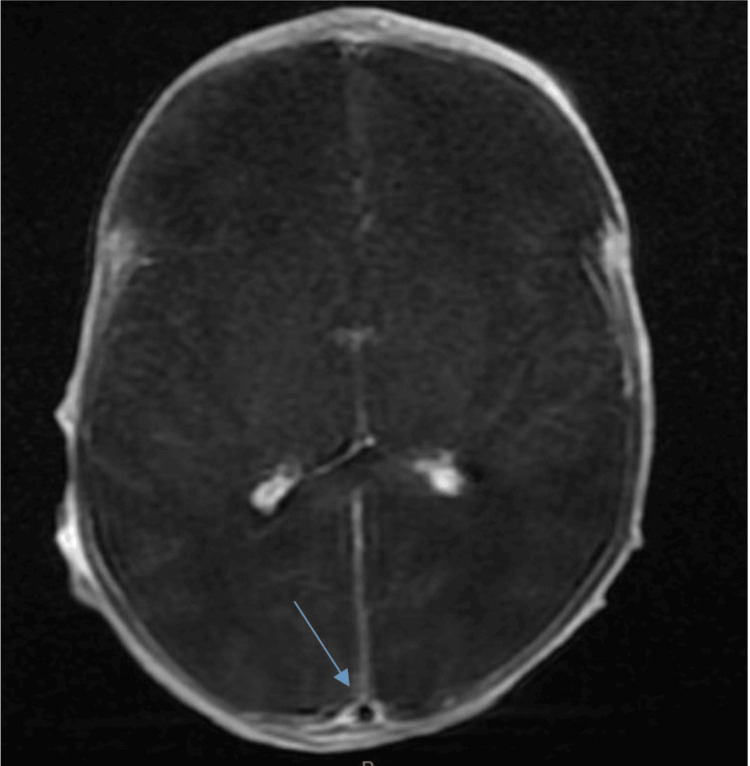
Brain MRI in axial T1 sequence with gadolinium injection showing the empty delta sign (blue arrow) MRI: magnetic resonance imaging
